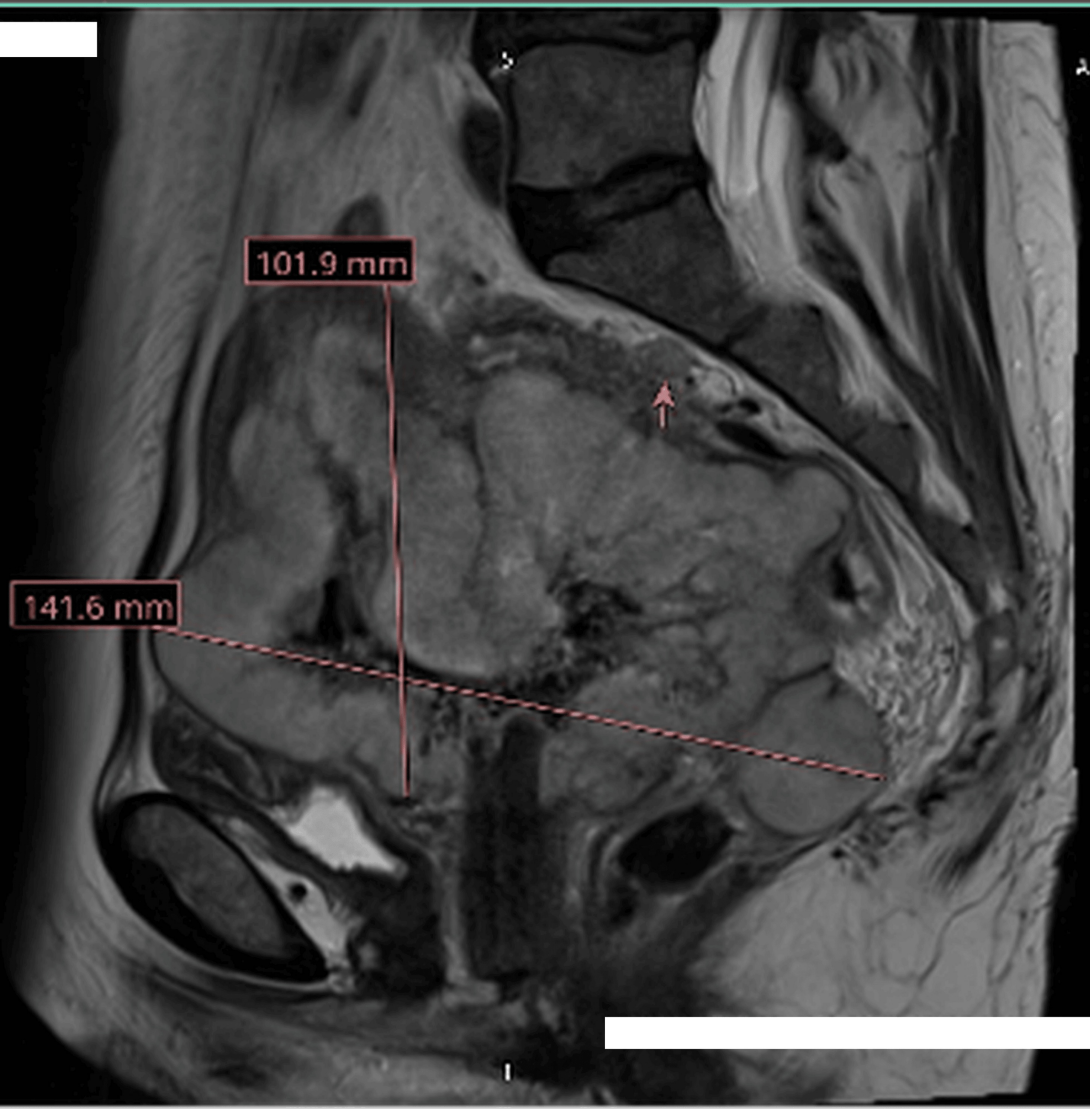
MRI pelvis showing a 10.1 cm x14.1 cm pelvic mass. The pink arrow is pointing towards a suspicious right obturator lymph node.
